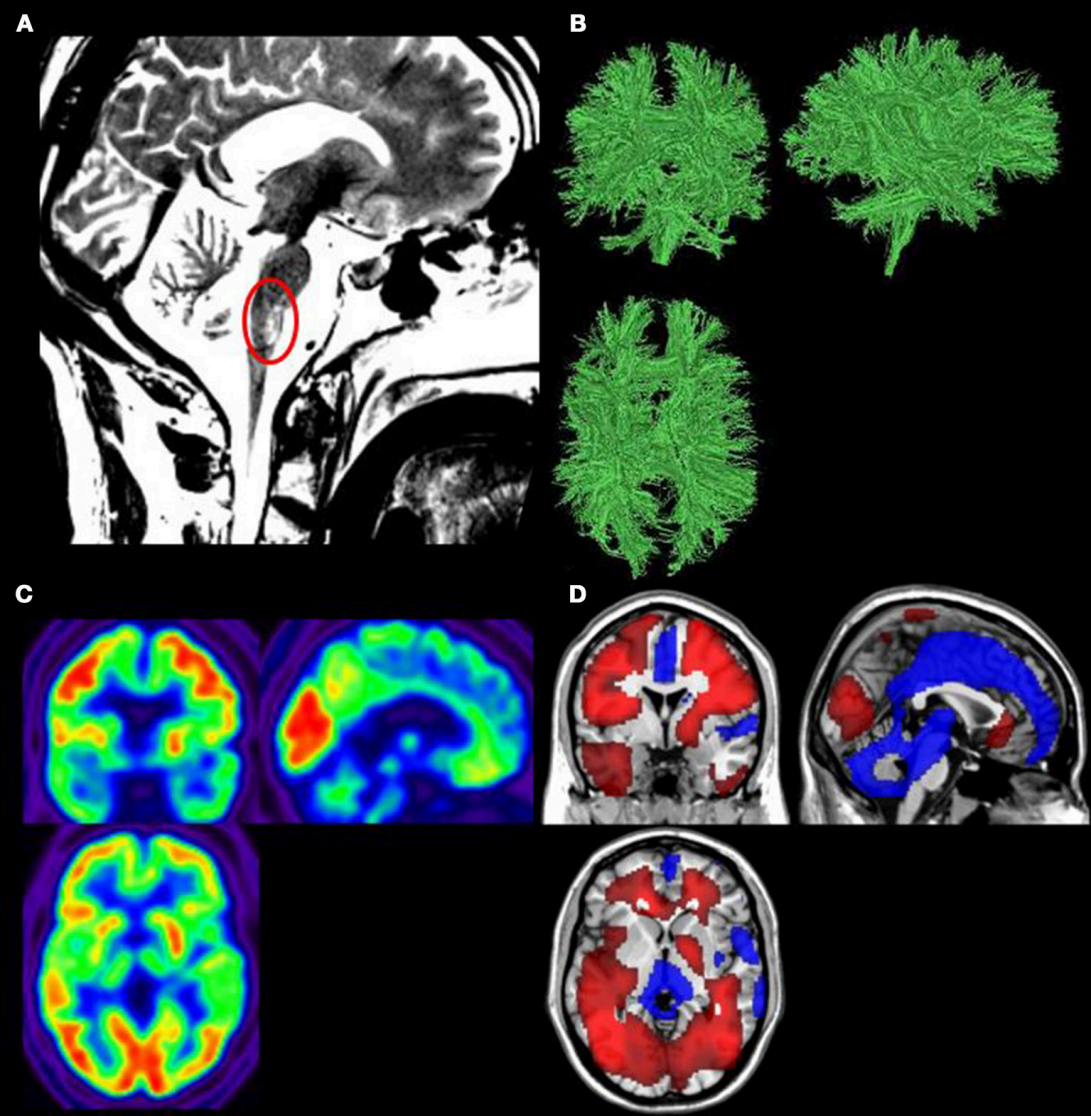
(A) Structural magnetic resonance imaging (MRI) showed the mesencephalic tegmentum lesion (red circle). (B) Diffusion tensor imaging (DTI) showed white matter structure preservation. (C)
